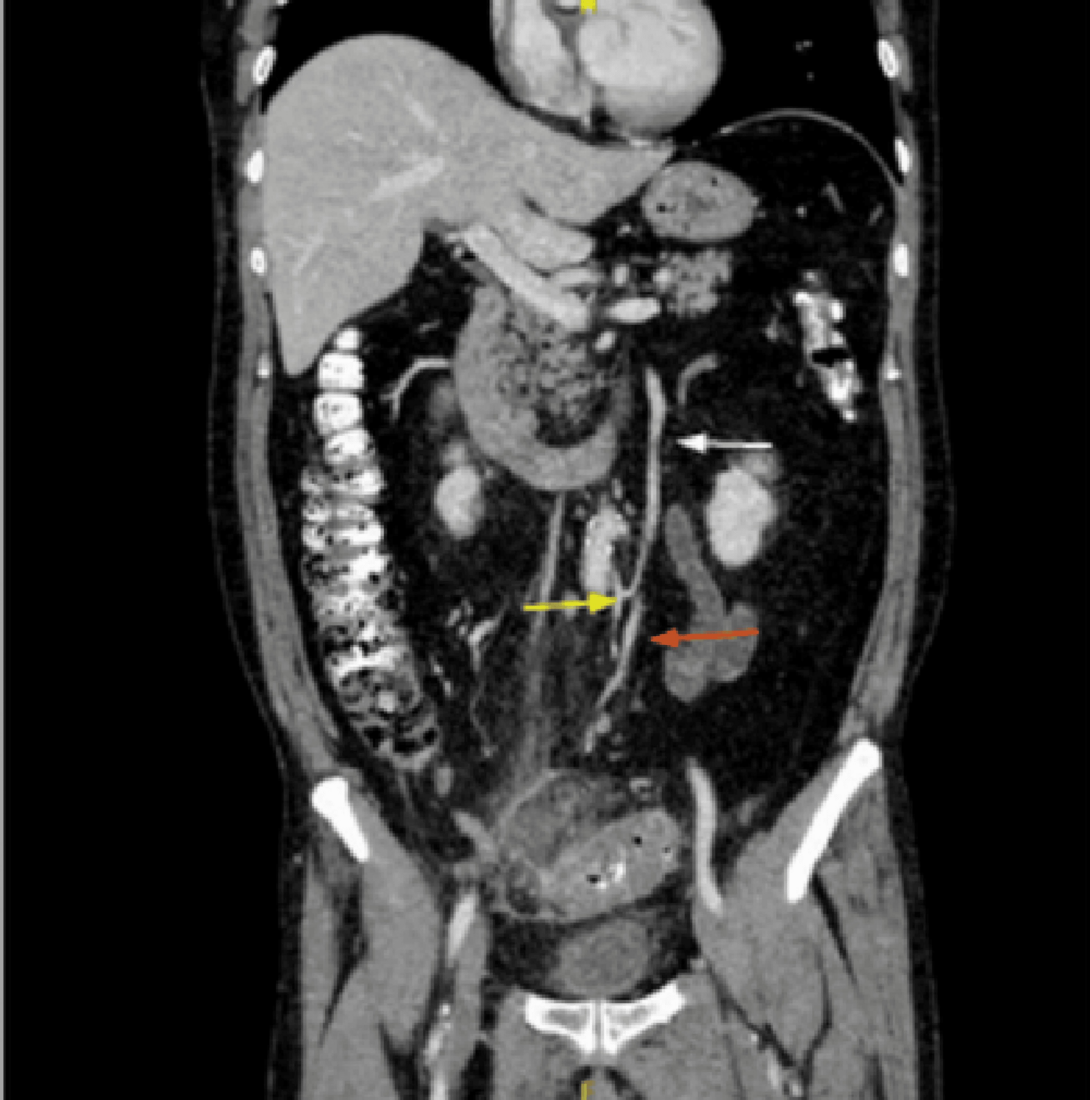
Coronal abdomen CT scan with contrast. White arrow indicates Moskowitz’s artery, orange arrow indicates inferior mesenteric vein, and yellow arrow indicates inferior mesenteric artery and its origin from aorta.
